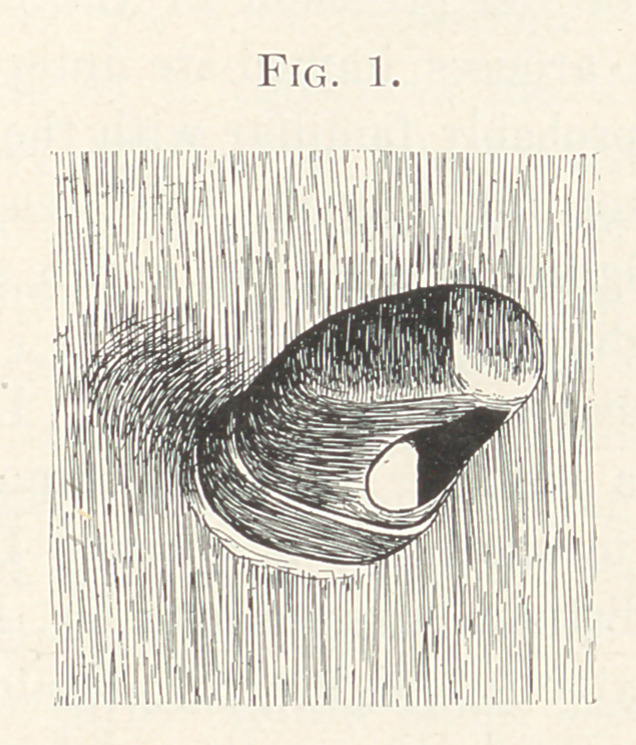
# The Therapeutic Use of the X-Ray in the Oral Cavity
